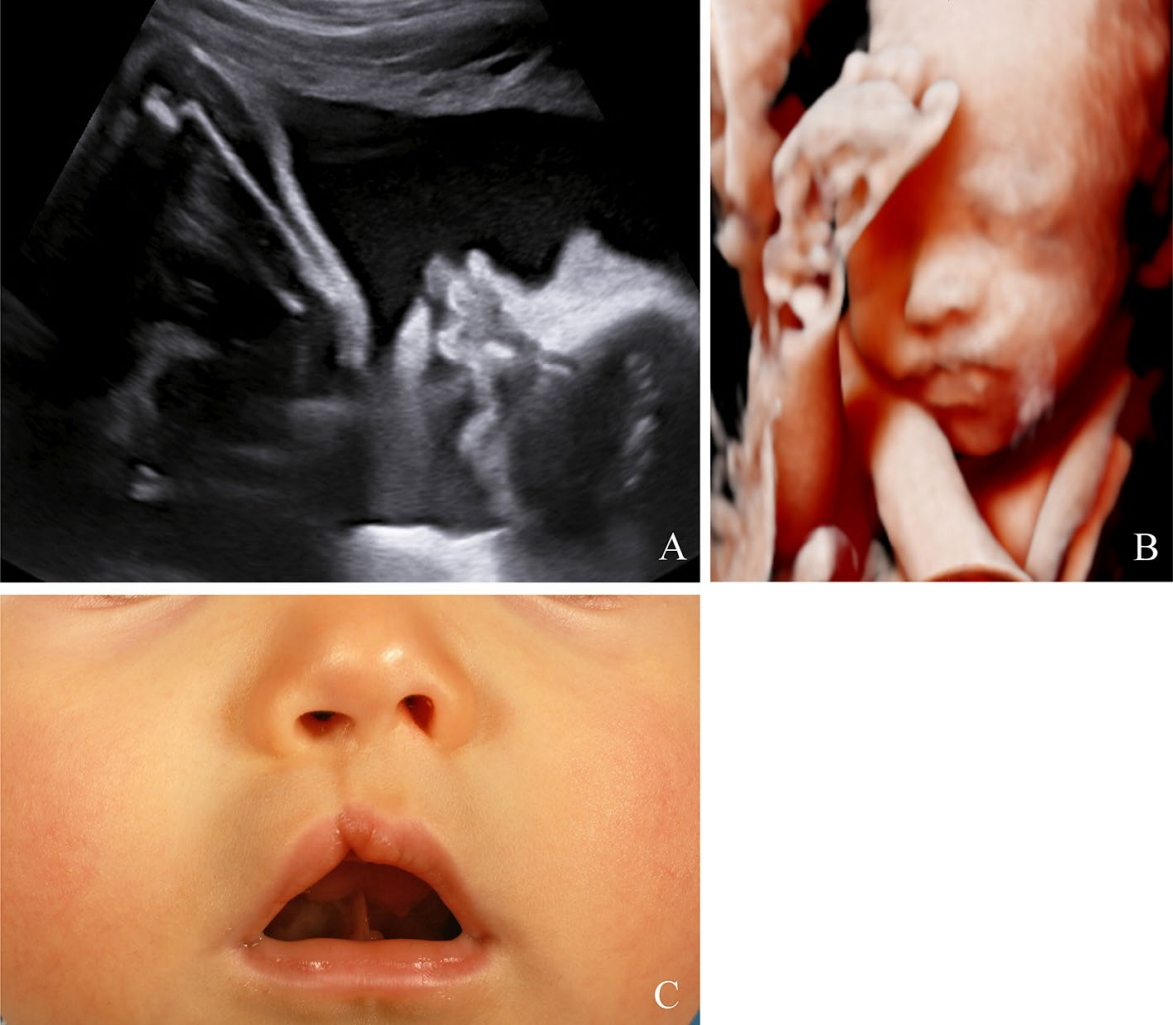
Unilateral cleft lip visualized on ultrasound during the prenatal period in 2D, in longitudinal section (A), and in 3D (B). Postnatal finding (C)
